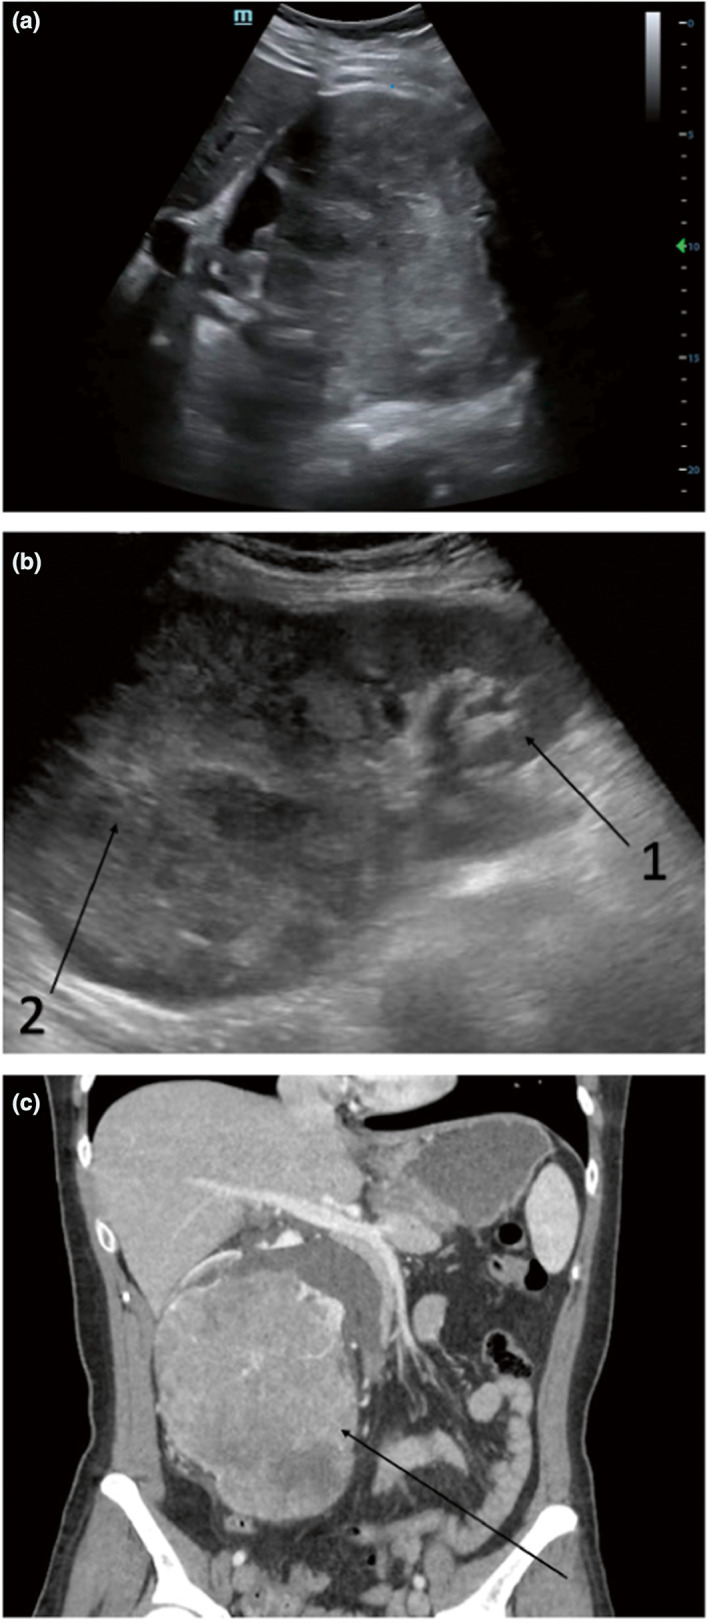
Renal cell carcinoma in the right kidney of a 50 year old woman. (a) Point‐of‐care ultrasound showing irregularity of the right renal cortex and obliteration of normal renal anatomy. (b) Radiology‐performed renal ultrasound showing a small area of remaining normal renal cortex and medulla (b1) as well as obliteration of superior renal cortex by an irregular hyperechoic mass (b2). (c) Coronal computed tomography abdomen/pelvis demonstrating right renal mass (arrow) ultimately diagnosed on pathology as renal cell carcinoma.
